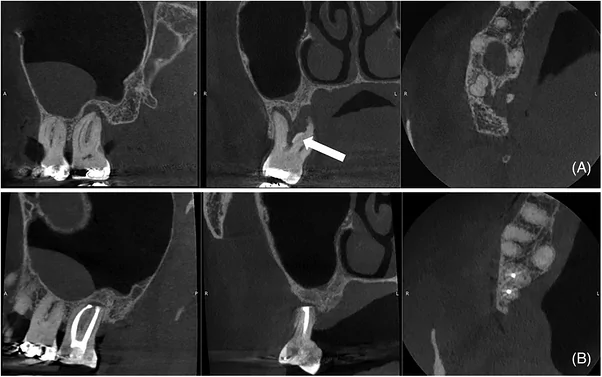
Case 1: Initial CBCT of tooth #2 (sagittal, coronal, axial), showing large hypodense lesion, dental calculus (arrow), furcation caries, and preserved buccal cortical (A). CBCT at 14 months postoperatively (sagittal, coronal, axial), showing bone formation on the distal surface of tooth #2 and apical and palatal remodeling of tooth #1. The hypodense line observed in the apical third of the buccal roots is consistent with repair tissue (collagenization) (B).
